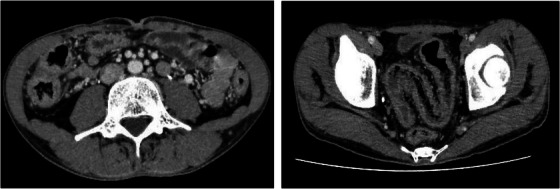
Contrast‐enhanced computed tomography revealed thickening of the colon and rectal wall and no small bowel lesions or vascular abnormalities.
